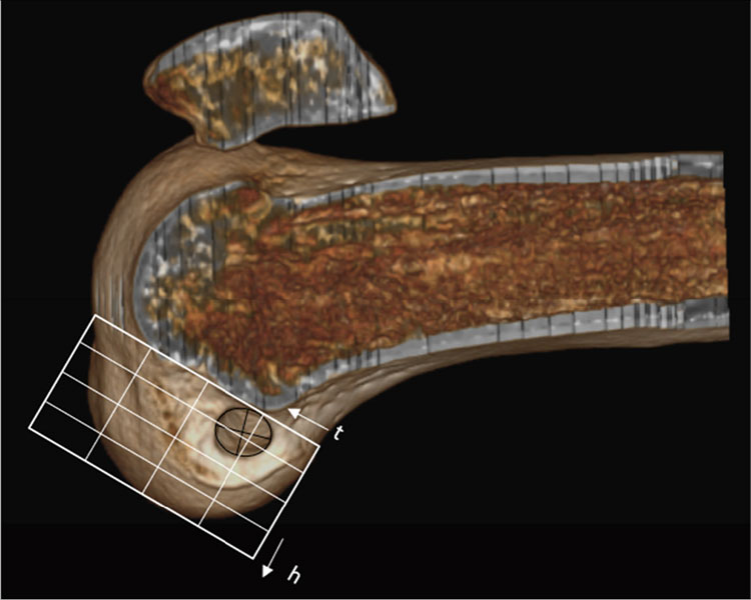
Evaluation with three‐dimensional computed tomography (CT)‐scanner with the medial femoral condyle removed and the knee in true sagittal view. A rectangular outline is created based on the Bernard quadrant method modified for 3D CT‐scanner. The horizontal limit corresponds to the total sagittal diameter of the lateral condyle along the Blumensaat line (distance *t*), measured as a percentage (0%–100%, deep/shallow). The vertical portion of the quadrant corresponds to the total distance from the intercondylar notch perpendicular to the Blumensaat line (distance *h*), also measured as a percentage (0%–100%, high/low). The location of the femoral tunnel within the quadrants is highlighted.
